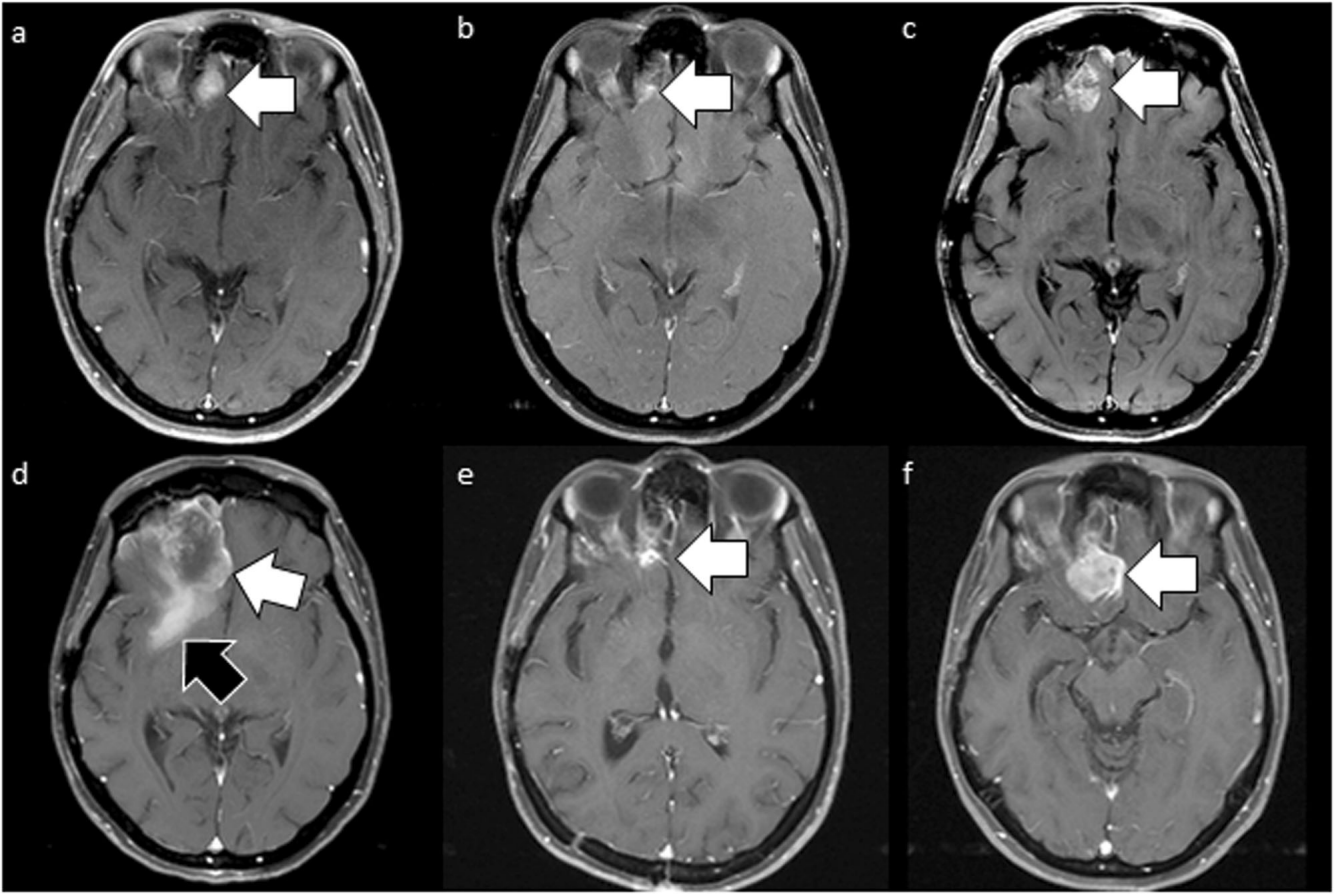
Radiological responses over time. a Axial contrast-enhanced MRI while the patient was on single-agent bevacizumab shows a dural-based frontal lobe metastasis (arrow). b Axial contrast-enhanced image 9 months after radiation therapy shows significant improvement in the irradiated lesion (arrow). c Axial contrast-enhanced MRI 5 months later shows recurrence of the metastasis (arrow). The lesion demonstrates heterogeneous enhancement. d Axial post-contrast MRI 2 months later shows the rapid growth of the cystic lesion (white arrow) and extensive perilesional enhancement (black arrow). e Axial post-contrast image at treatment nadir 2 months after dabrafenib shows a significant decrease in the size of the lesion. f Axial post-contrast image 3 months later shows recurrence of the lesion, this time with solid enhancement (white arrow). The lesion was subsequently resected, showing gliosarcoma.
